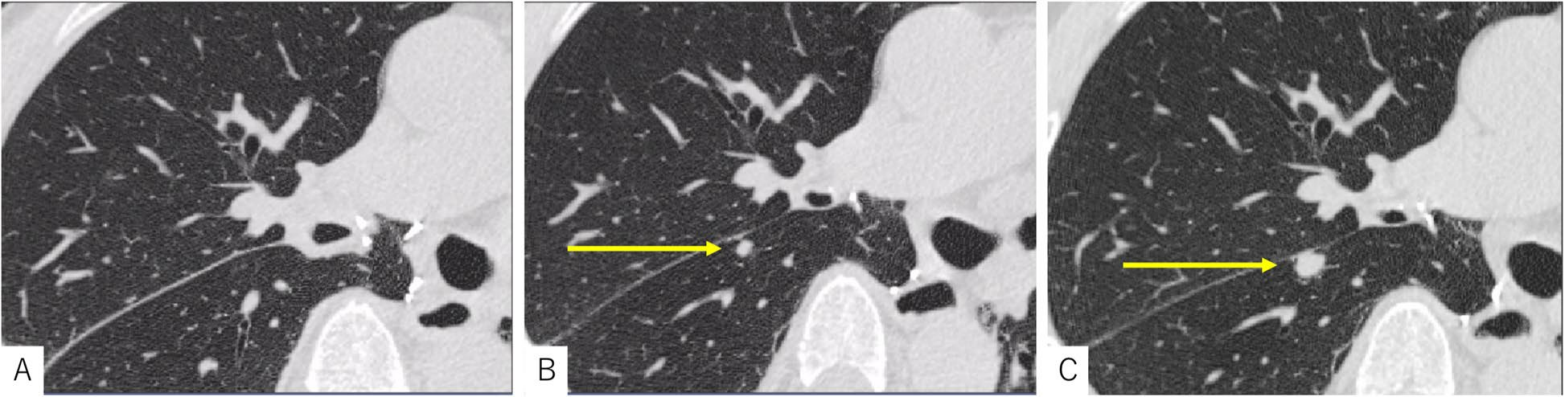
Changes in the pulmonary nodule on chest computed tomography. A Three months after right single-lung transplantation, the nodule was hardly identifiable. B The nodule was first recognized 1 year after right single-lung transplantation (arrow). C At 18 months after lung transplantation, the nodule was enlarged (arrow)
